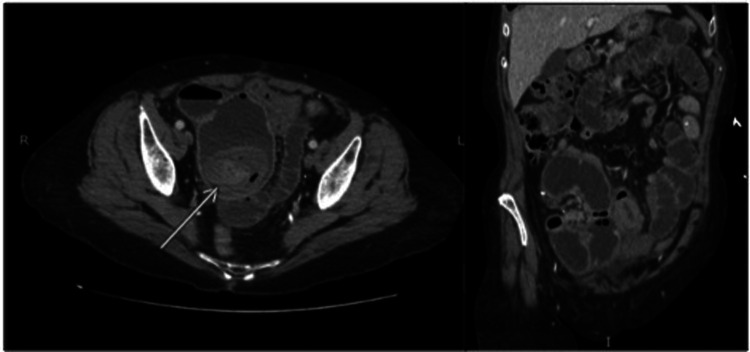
Preoperative abdominal computed tomography. Preoperative abdominal computed tomography demonstrating air fluid levels and dilated loops of small bowel. The findings were concerning for small bowel obstruction due to intussusception and localized perforation.
